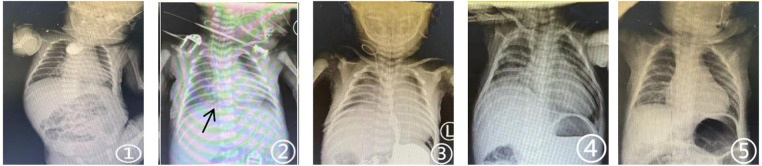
Serial chest x-rays of case 1 (conservative management). (1) (Preoperative): Esophagogram showing the proximal esophageal pouch. (2) (Postoperative day 3): Bedside chest x-ray showing the chest tube positioned in the right paravertebral mediastinum (arrow) with normal diaphragmatic contour. (3) (Postoperative day 7): Bedside chest x-ray revealing significant elevation of the right hemidiaphragm. (4) (Postoperative day 42): Chest x-ray showing persistent right hemidiaphragm elevation. (5) (1-month follow-up): Chest x-ray demonstrating the right hemidiaphragm largely returned to its anatomical position.
